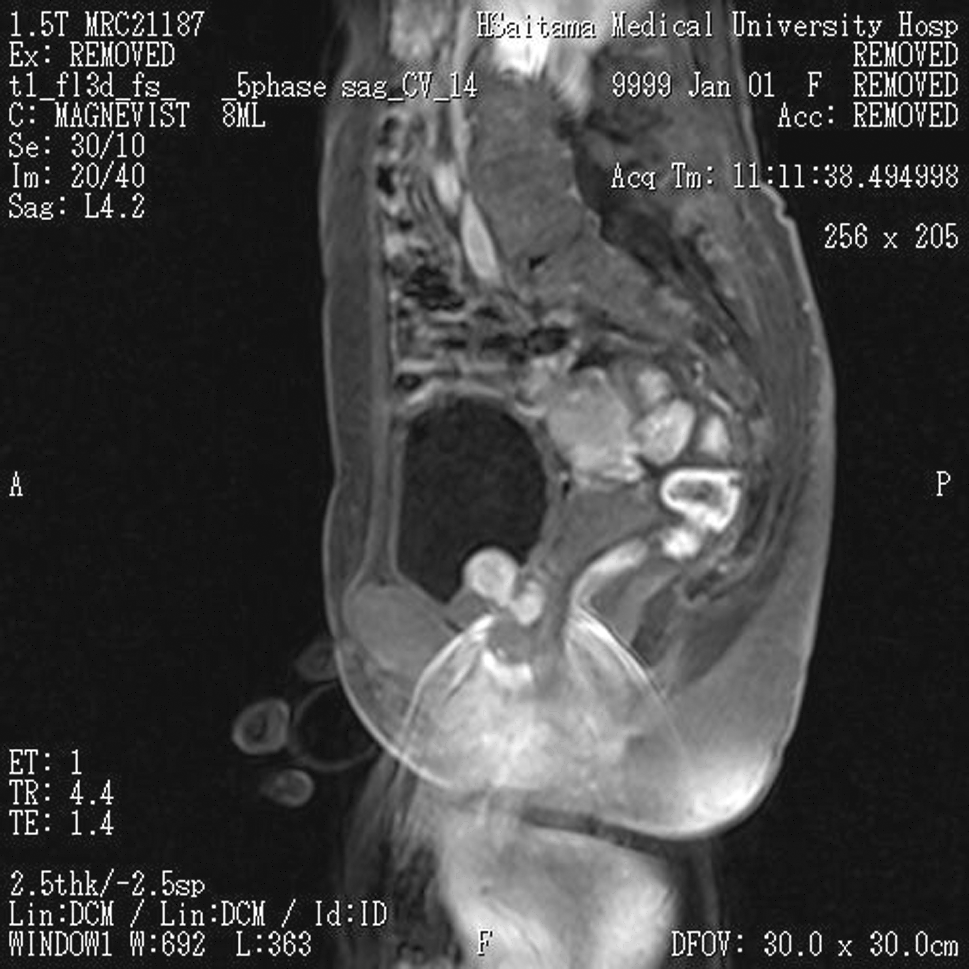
Sagittal section of a magnetic resonance image. Sagittal section of a magnetic resonance image shows a homogeneous mass (2.7 cm in diameter) occupying the area between the bladder neck and the anterior vaginal wall. The tumor mass was homogenously enhanced after the injection of gadolinium.
